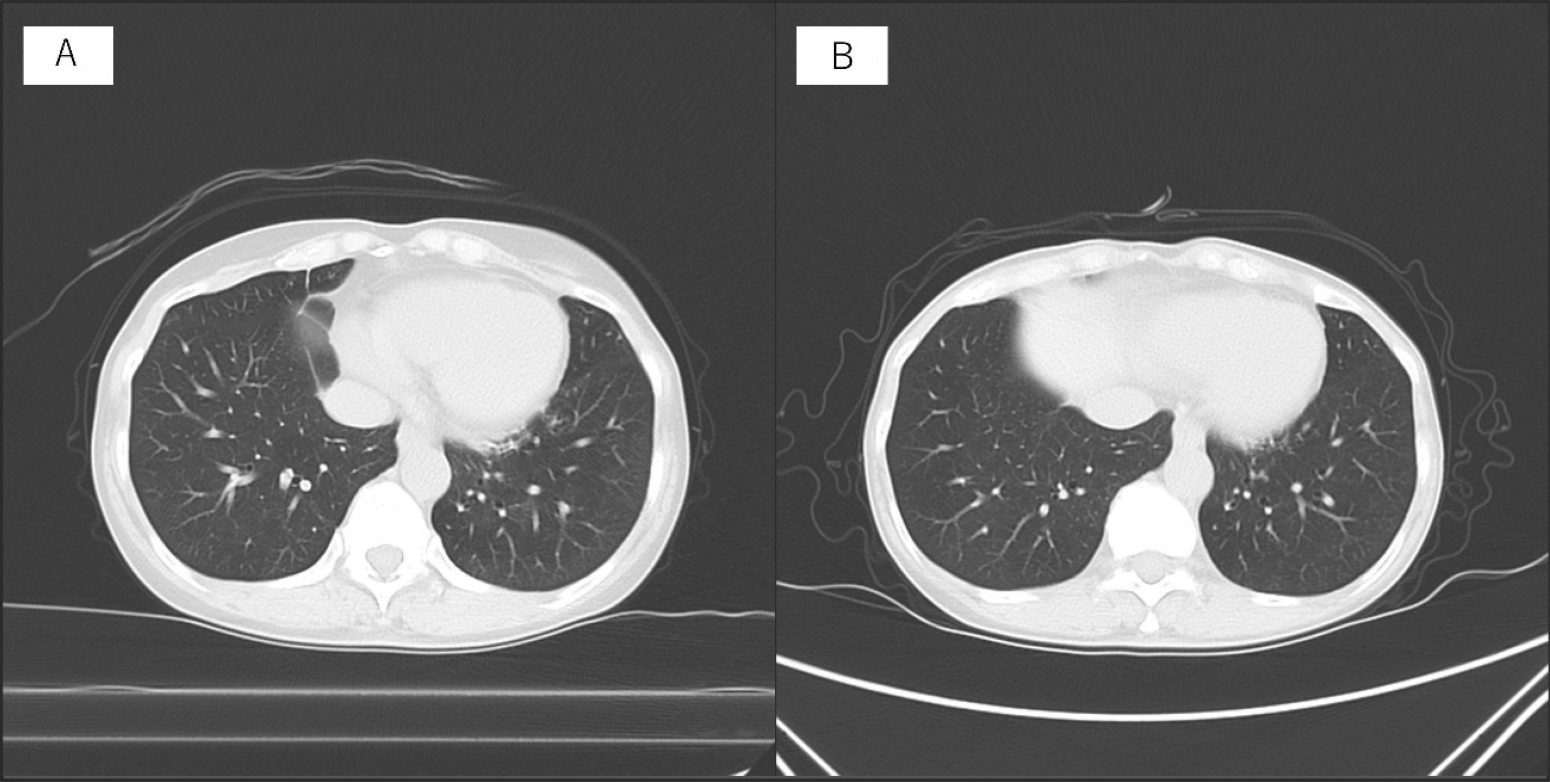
CT scans before COVID‐19. (A) Chest CT scan at 5.5 years before COVID‐19. (B) Chest CT scan at one half year before COVID‐19. No abnormal findings were observed.
